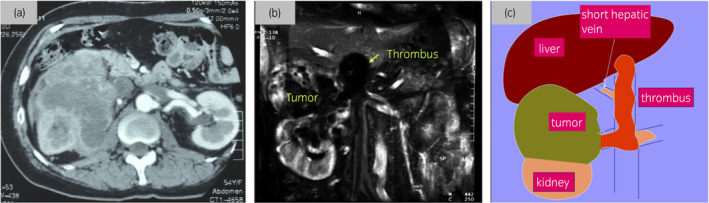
(a) Abdominal computerized tomography (CT) revealed a heterogeneously enhancing solid mass in the right kidney and a thrombus in the IVC. (b) Magnetic resonance imaging (T2‐weighted) reveals tumor of slightly high signal intensity in the right kidney and thrombus extending into renal vein and vena cava. (c) Shema of renal tumor and vena cava thrombus. The thrombus extended in short hepatic vein and left renal vein
